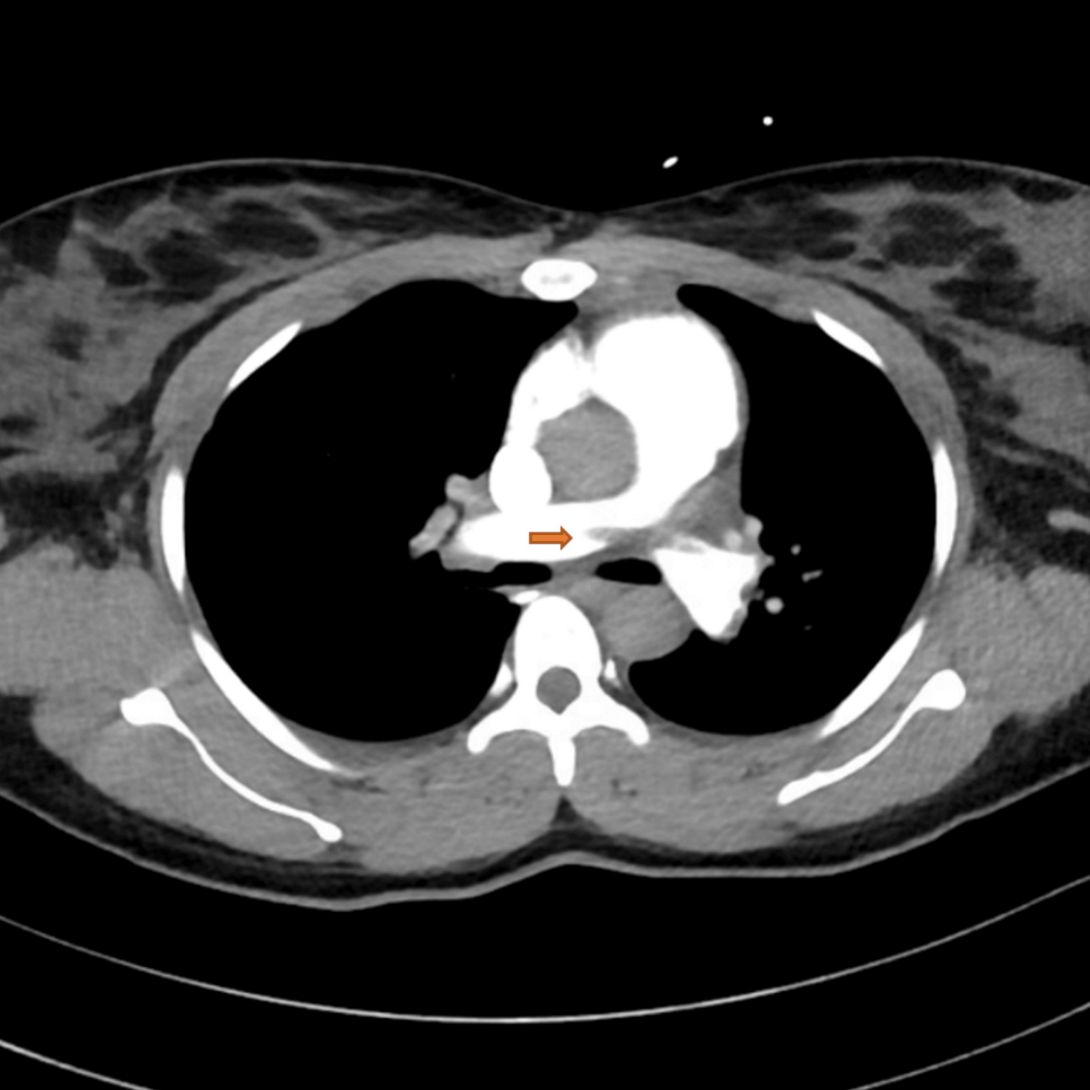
Computed tomography pulmonary angiography (CTPA) showing the extension of the saddle embolus at the pulmonary trunk bifurcation.
